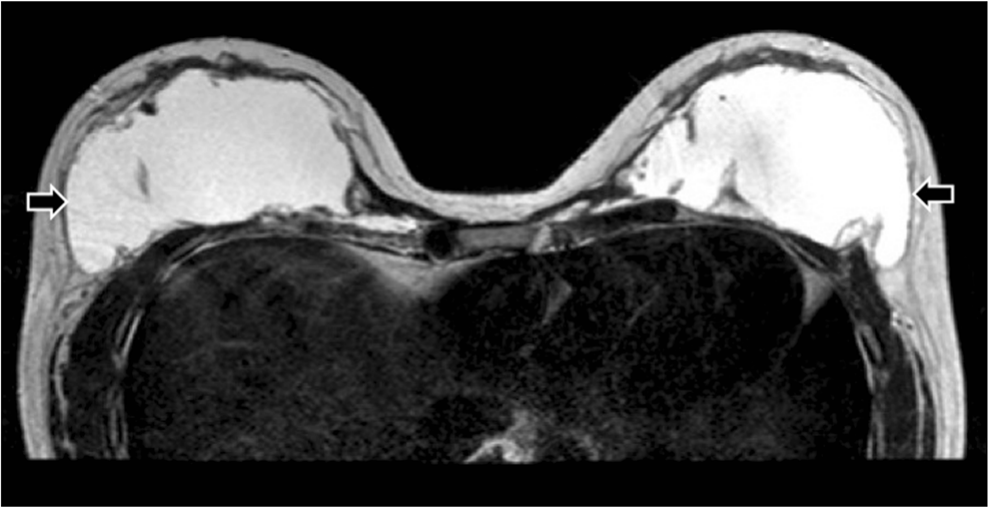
Desirable retroglandular position of PAAG. T2-weighted axial MR image demonstrates uncomplicated PAAG injection, with a collection of homogeneous T2 hyperintense material (arrows) at the retroglandular region of each breast, located anterior to the pectoral muscles
